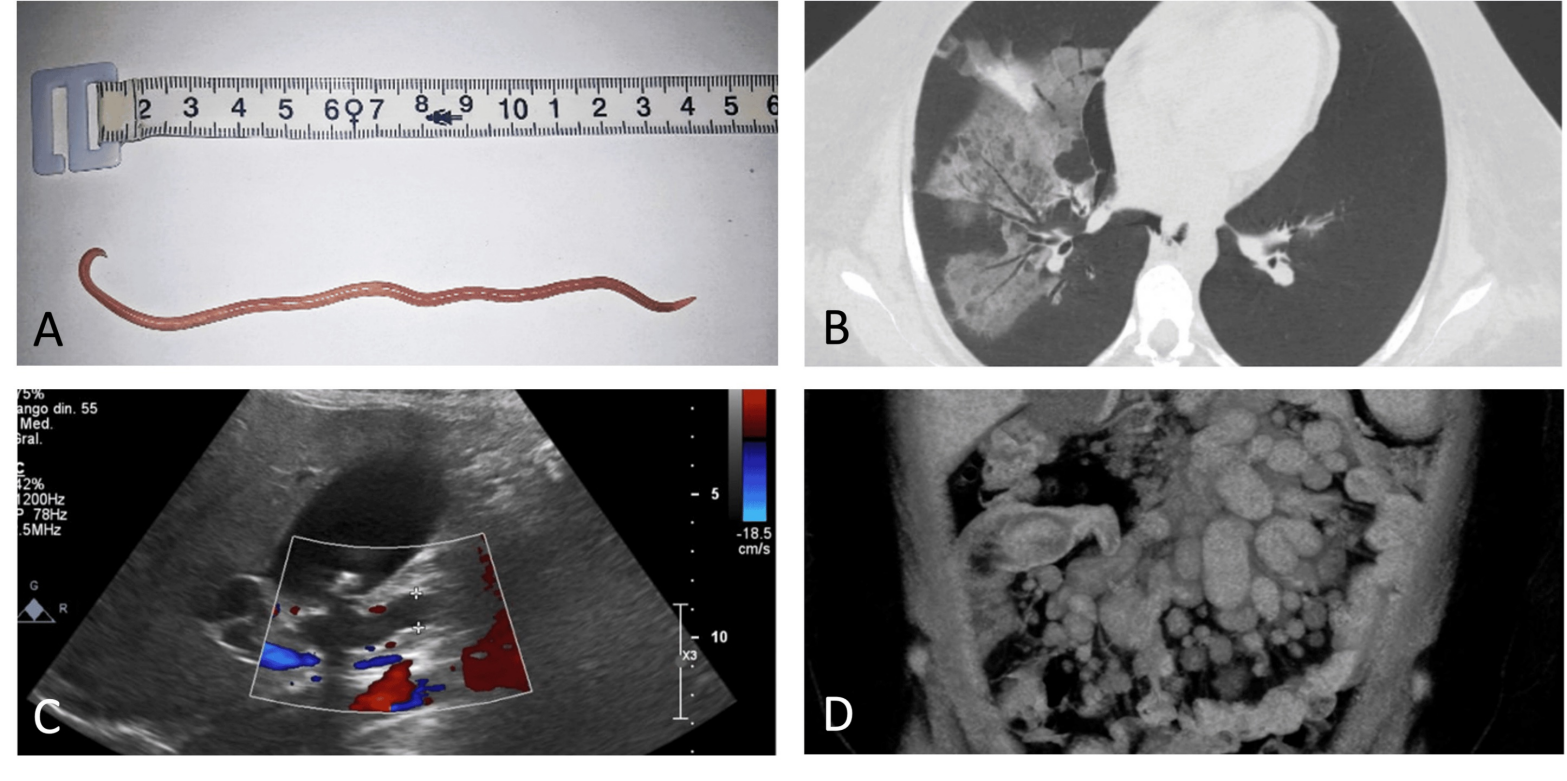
Multisystemic findings (A) Expelled adult Ascaris lumbricoides worm measuring ~15 cm. (B) Chest CT showing ground-glass opacities and mosaic “head cheese” pattern. (C) Doppler ultrasound showing dilation of intrahepatic and extrahepatic bile ducts. (D) Abdominal CT demonstrating mesenteric lymphadenopathy and ileo-ileal intussusception CT: computed tomography
